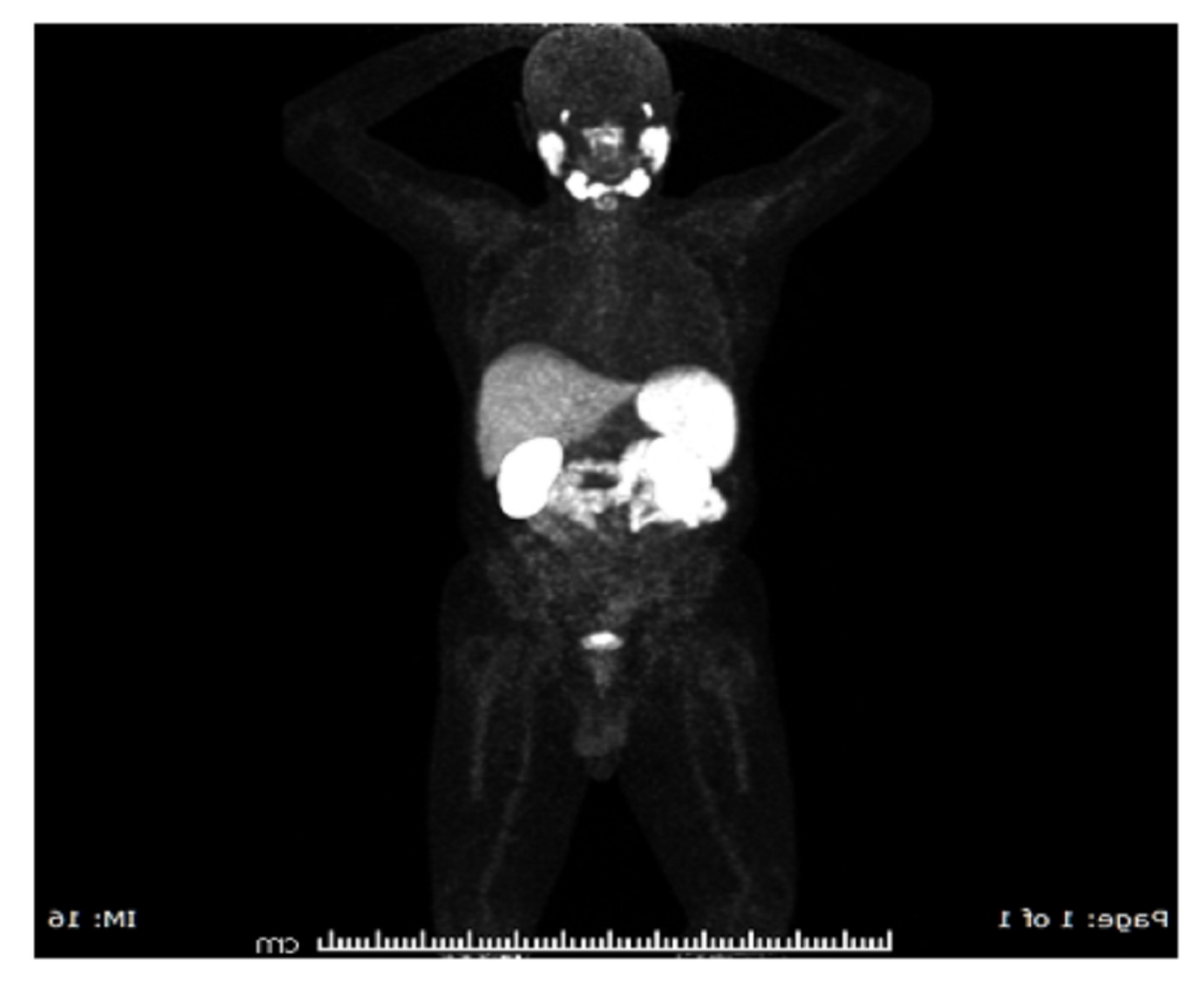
PSMA PET-CT coronal MIP image shows no detected tumor recurrence in the prostate, lymph nodes, or distant metastasis. PSMA: prostate-specific membrane antigen; PET-CT: positron emission tomography-computed tomography; MIP: maximum intensity projection
